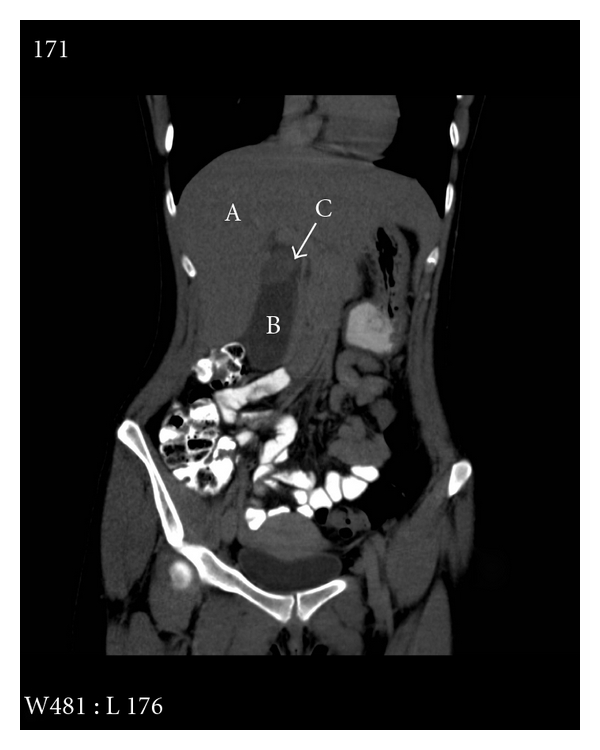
CT scan of Abdomen and Pelvis with soft tissue mass in proximity to the posterior wall of the gallbladder. A: liver, B: body of Gallbladder, and C: soft tissue mass.
